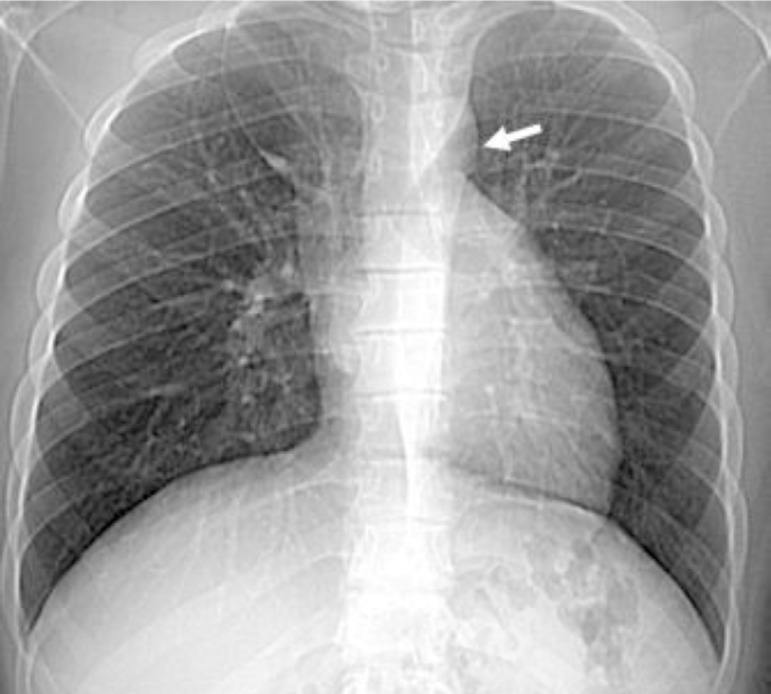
CT scout view was showing a lucent area between the aorta and pulmonary
